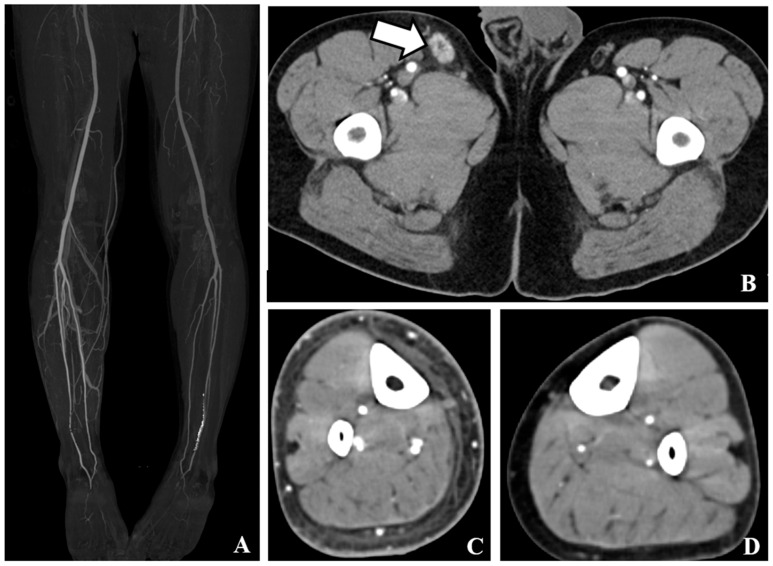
CT findings of cellulitis. (A). Maximum intensity projection images show early venous return at right lower limb. (B). Contrast-enhanced axial computed tomography (CT) showed prominent inguinal lymph node enhancement with enlargement of the right inguinal area. Slight perinodal fat infiltration is noted (white arrows). (C). Contrast-enhanced axial CT tomography revealed diffuse skin thickening and subcutaneous edema in the right lower leg. (D). Contrast-enhanced axial CT scan shows no abnormal finding at left lower leg.
